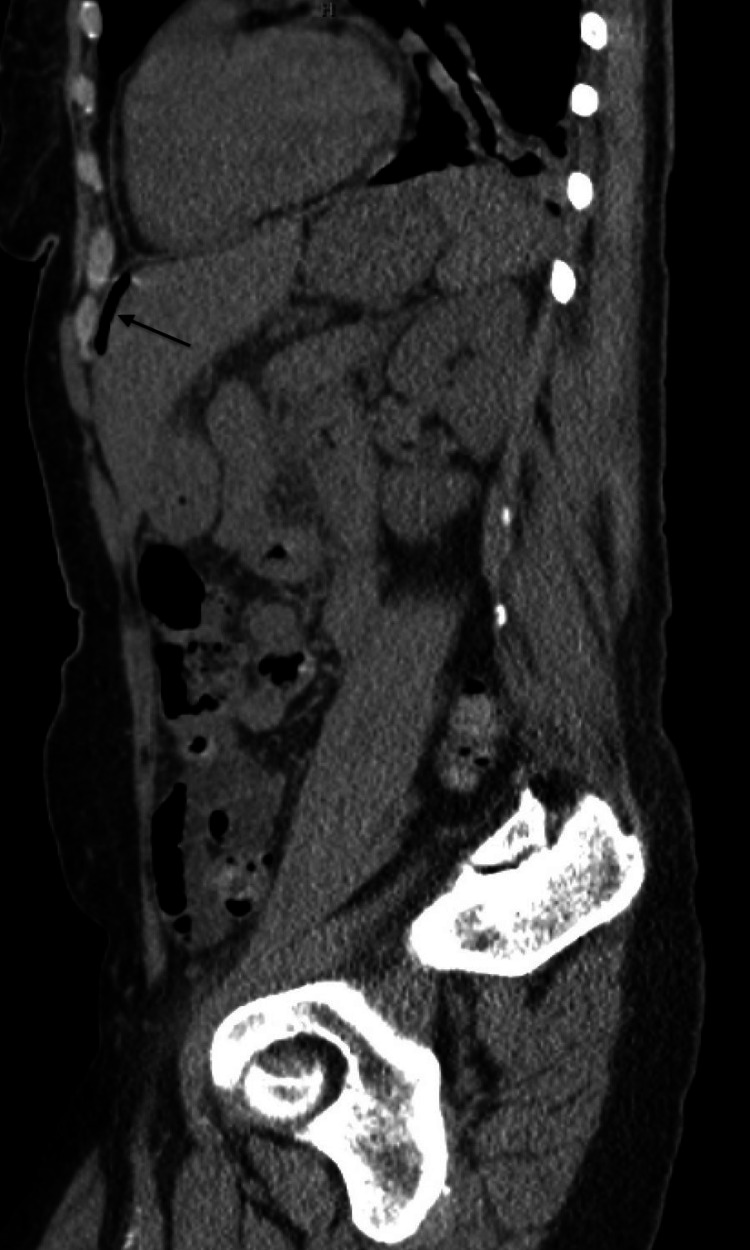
computed tomography sagittal image Pneumoperitoneum (arrows)
